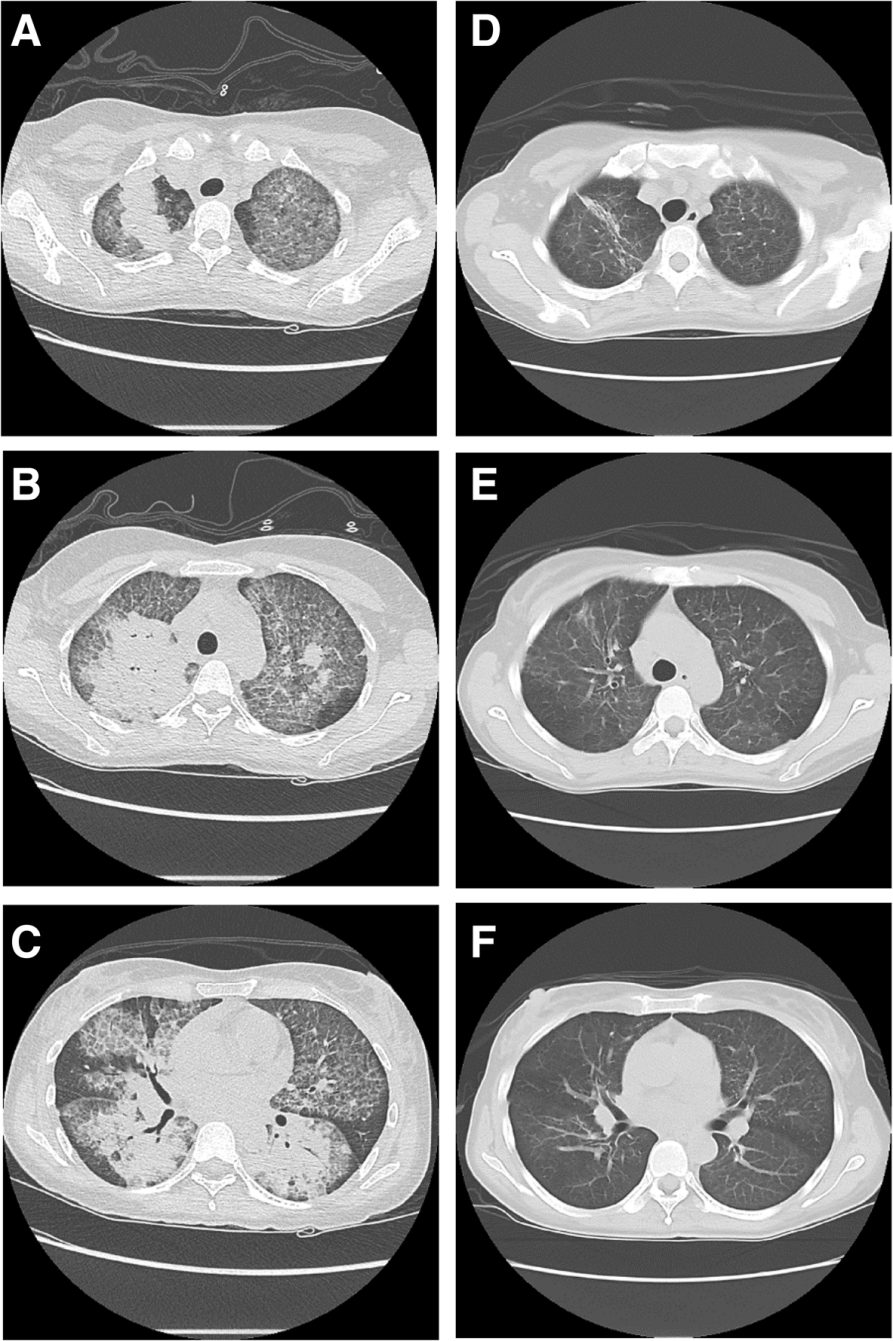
Noncontrast CT chest on presentation and following treatment. a-c: representative cross-sectional images of the chest on presentation. d-f: matched images following completion of combined-modality therapy with GM-CSF and serial lobar lavage
